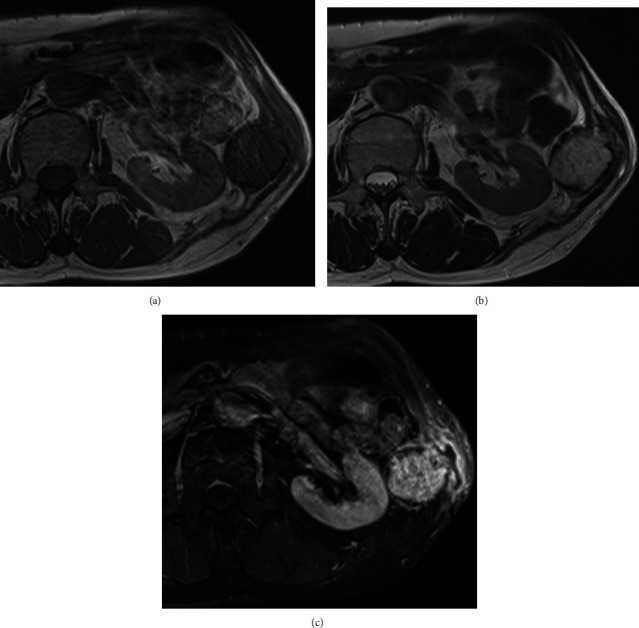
Magnetic resonance imaging showing the tumor in the 11th rib. (a) The mass was isointense relative to skeletal muscle on T1-weighted images. (b) The mass was hyperintense relative to skeletal muscle on T2-weighted images. (c) The mass showed enhancement on gadolinium-enhanced, fat-saturation T1-weighted images.
